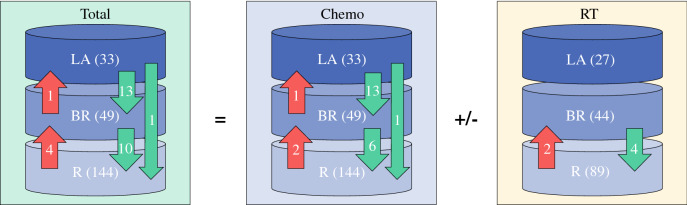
Changes in radiographic stage that occurred in association with preoperative therapy. *Chemo* chemotherapy, *RT* radiation therapy, *LA* locally advanced, *BR* borderline resectable, *R* resectable
